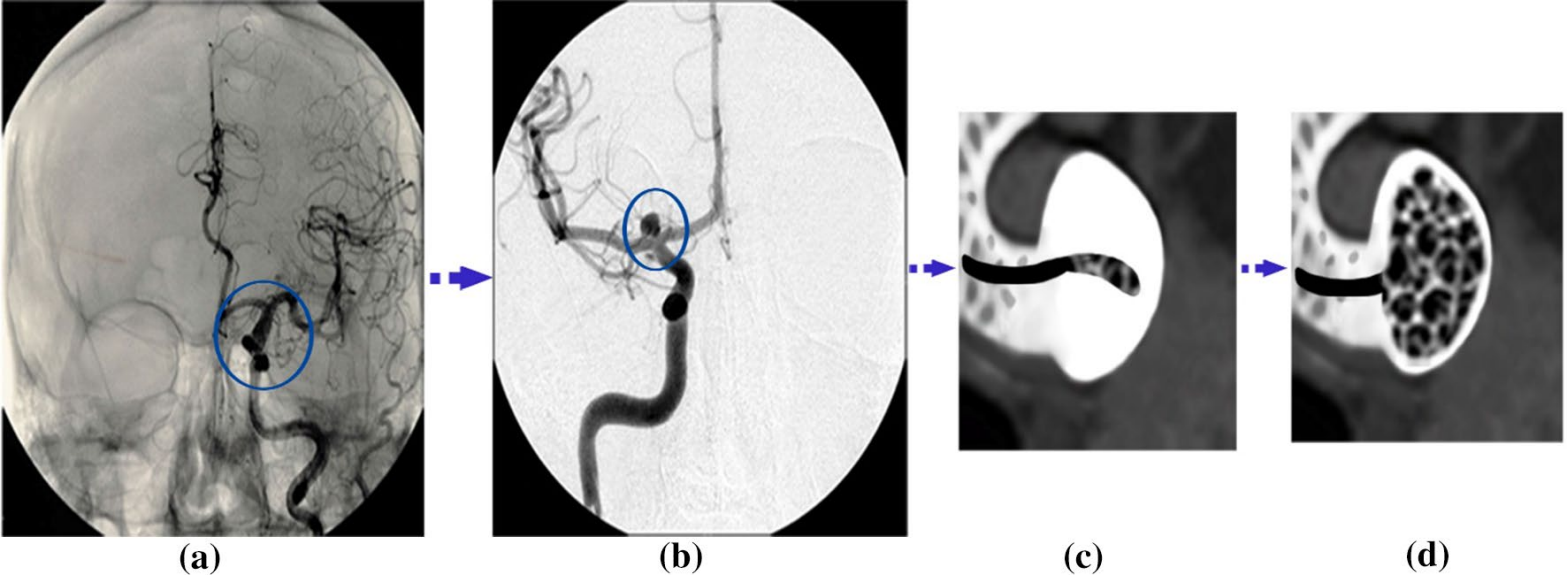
The process of treatment with SMPUFs: a reorganization of aneurysm with CT scan, b estimation of aneurysm size, c crimped (temporary shape) SMP foam device delivered to the aneurysm via catheter, d expansion of SMPUF within the aneurysm, as it recovers to its permanent shape via stimuli
